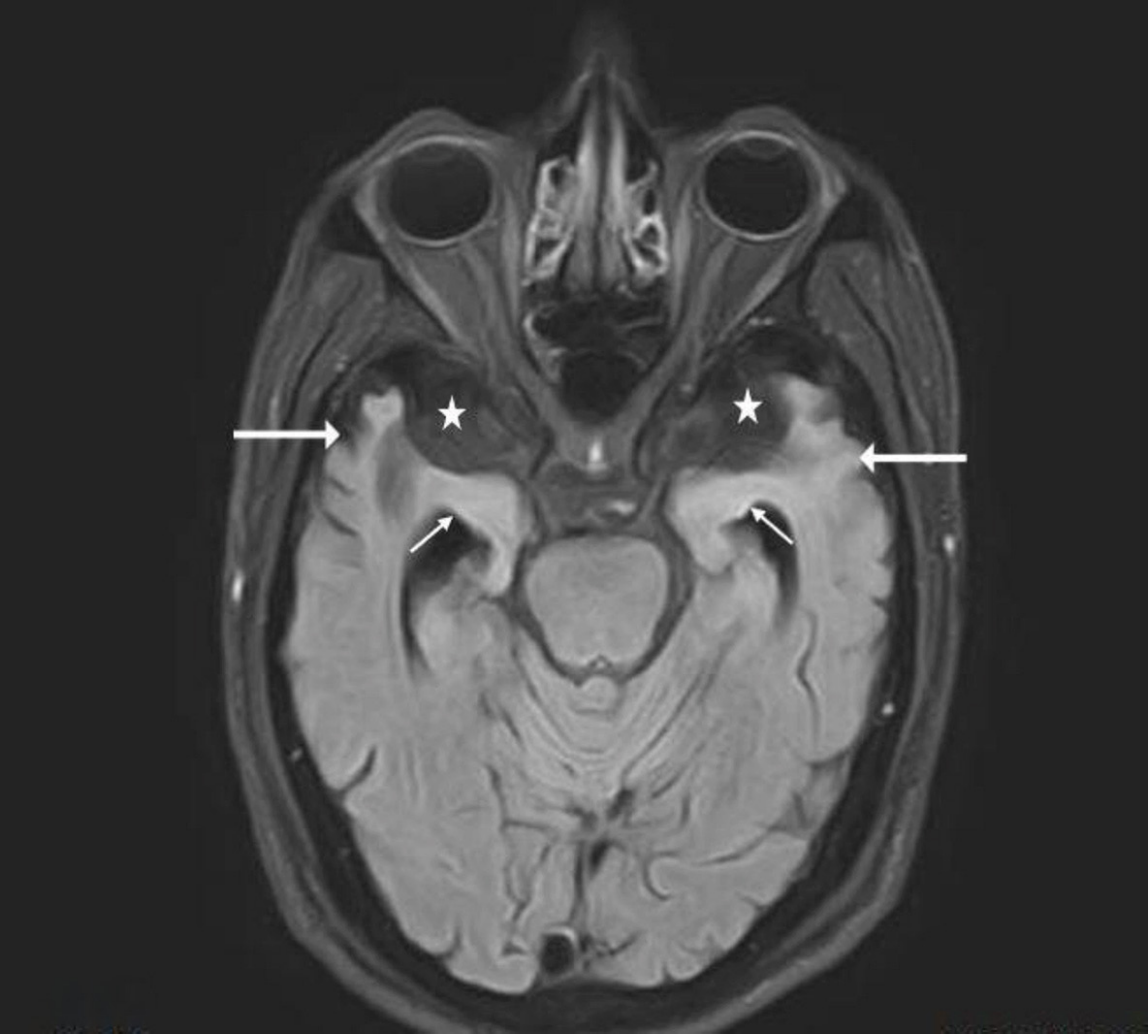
Axial FLAIR sequence of the brain at the level of the midbrain showing dilated extra-axial CSF spaces in the middle cranial fossa anteriorly (white asterisks), along with ex vacuo dilatation of the temporal horns of both lateral ventricles (short white arrow) with atrophy of both temporal lobes (long white arrows). FLAIR: Fluid atenuated inversion recovery.
